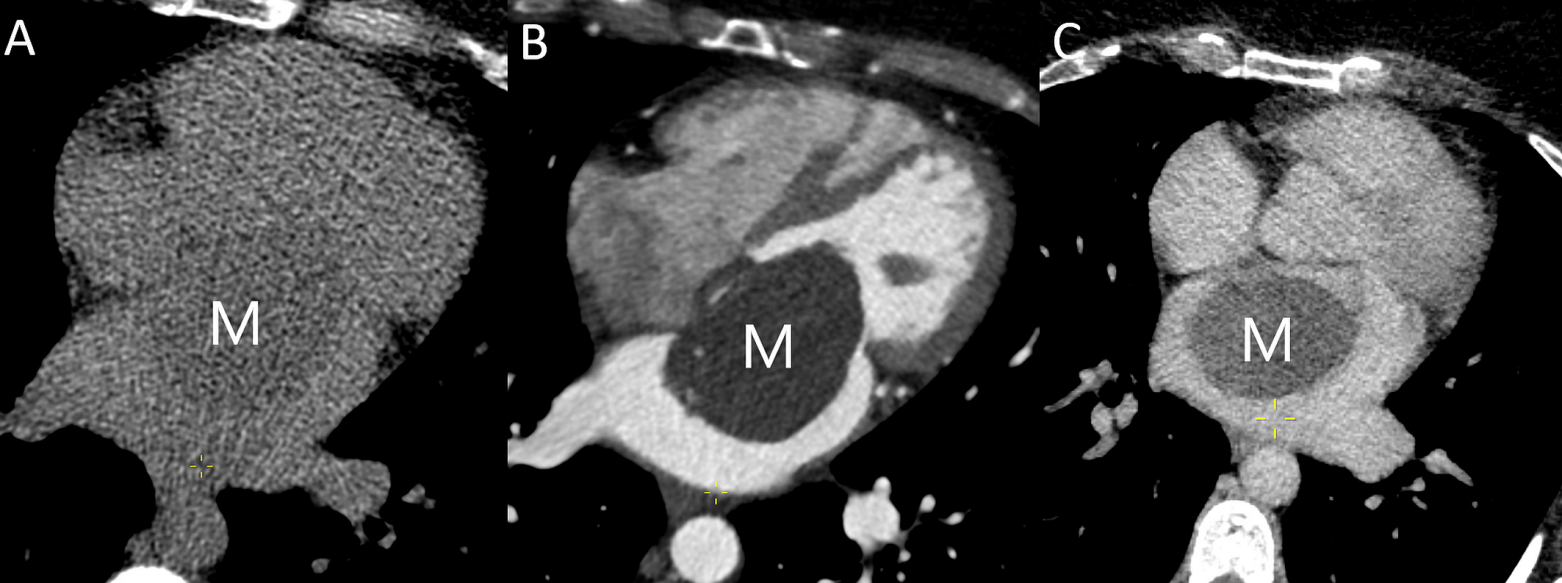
Left atrial myxoma (M) imaged using cardiac CT. (A)
